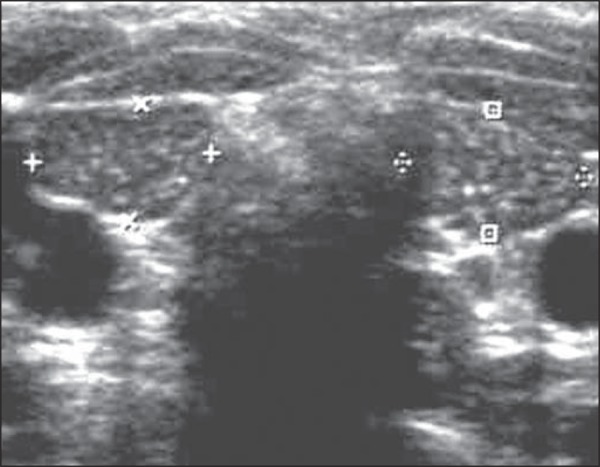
Ultrasonography of cervical region. On this image, the thyroid is hyperechogenic
